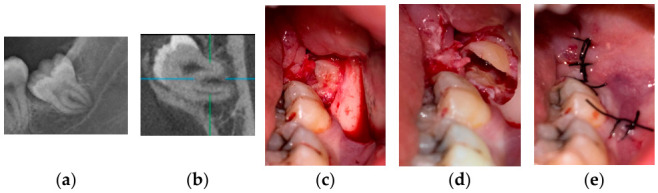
A qualitative comparison and the surgical stages of a “Plan Change” case: (a) An OPG view showing the apparent superimposition of the MM3 roots and IAC, initially categorized as high-risk. (b) The corresponding CBCT cross-section of the same tooth revealing a safe lingual distance and intact cortical border, leading to a modification in the surgical plan. (c) The surgical area after flap elevation and clinical sight of the impacted MM3. (d) Tooth sectioning and buccal bone removal. (e) Surgery area after extraction and wound sutured with a 3/0 silk suture.
